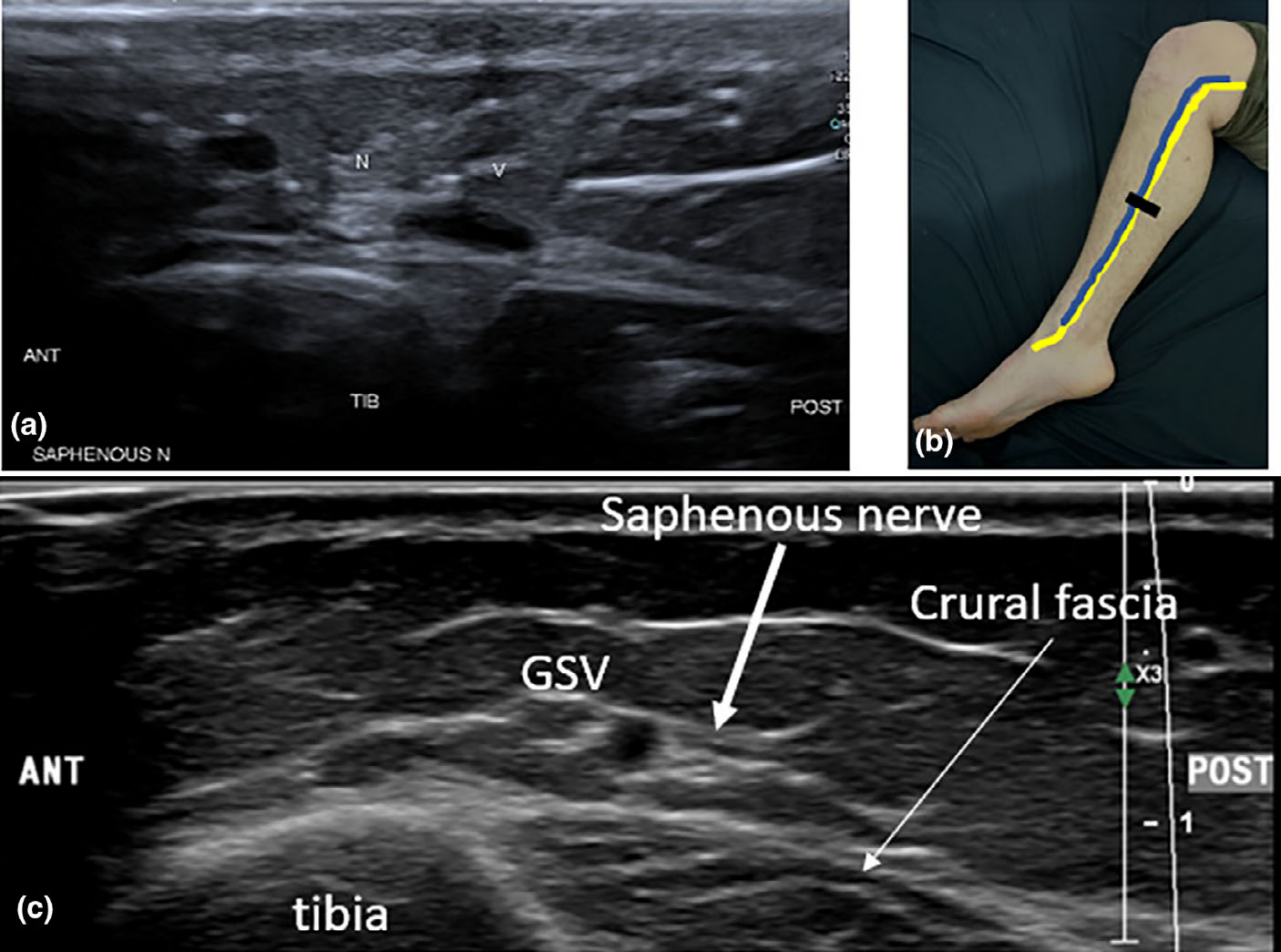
Saphenous nerve in the lower leg. (a) The saphenous nerve (N) can be in variable positions relative to the great saphenous vein (GSV). In this short‐axis sonographic image, the saphenous nerve is anterior to the GSV. It overlies the edge of the tibia (TIB). (b) The position of the saphenous nerve (yellow), which follows the path of the GSV (blue). Transducer position mid‐leg is demonstrated by the black line for short‐axis imaging of the vein and nerve at the mid‐lower leg region, corresponding to image (a). (c) Short‐axis sonographic image demonstrating the saphenous neve (thick white arrow) posterior to the GSV. Both sit superficial to the crural fascia (thin white arrow). ANT, anterior aspect of image; POST, posterior aspect of image.
